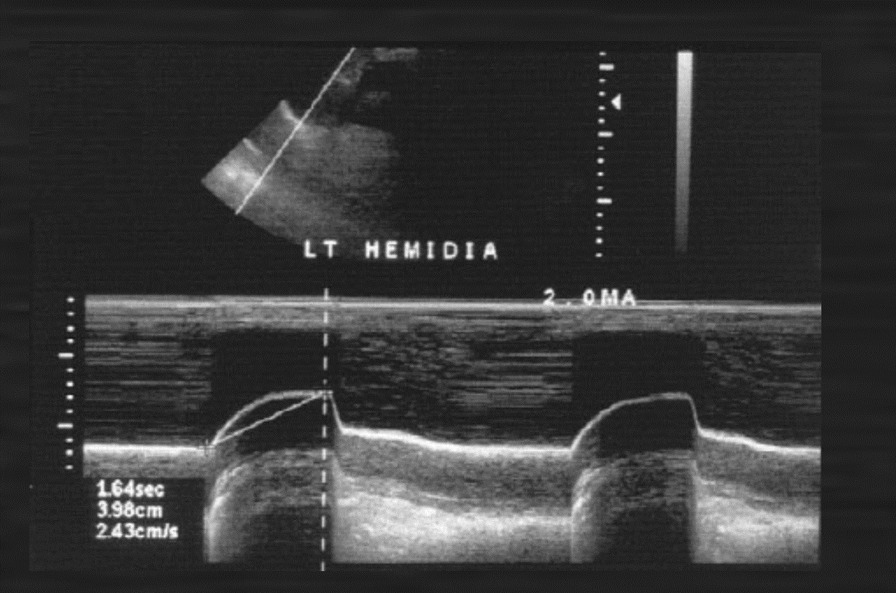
Paralyzed left hemidiaphragm with implanted electronic pacer. M-mode imaging shows diaphragm motion in response to the pacer triggering. Reproduced under Open Access Creative Commons License: Gerscovich et al. J Ultrasound Med 2001;20(6):597–604
